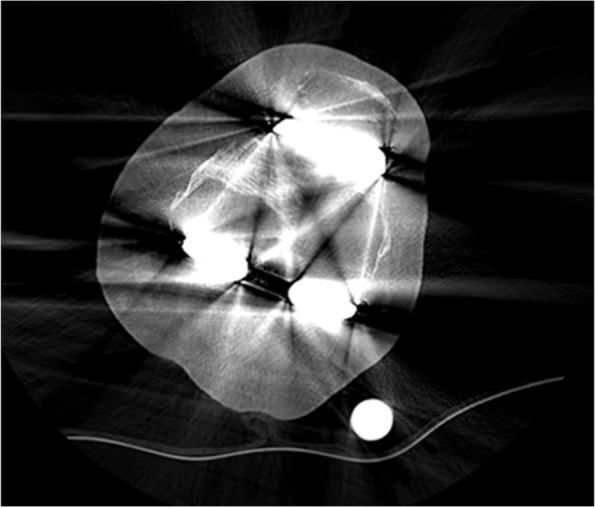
CT slice of a femoral component without metal artefact reduction software (MARS)
